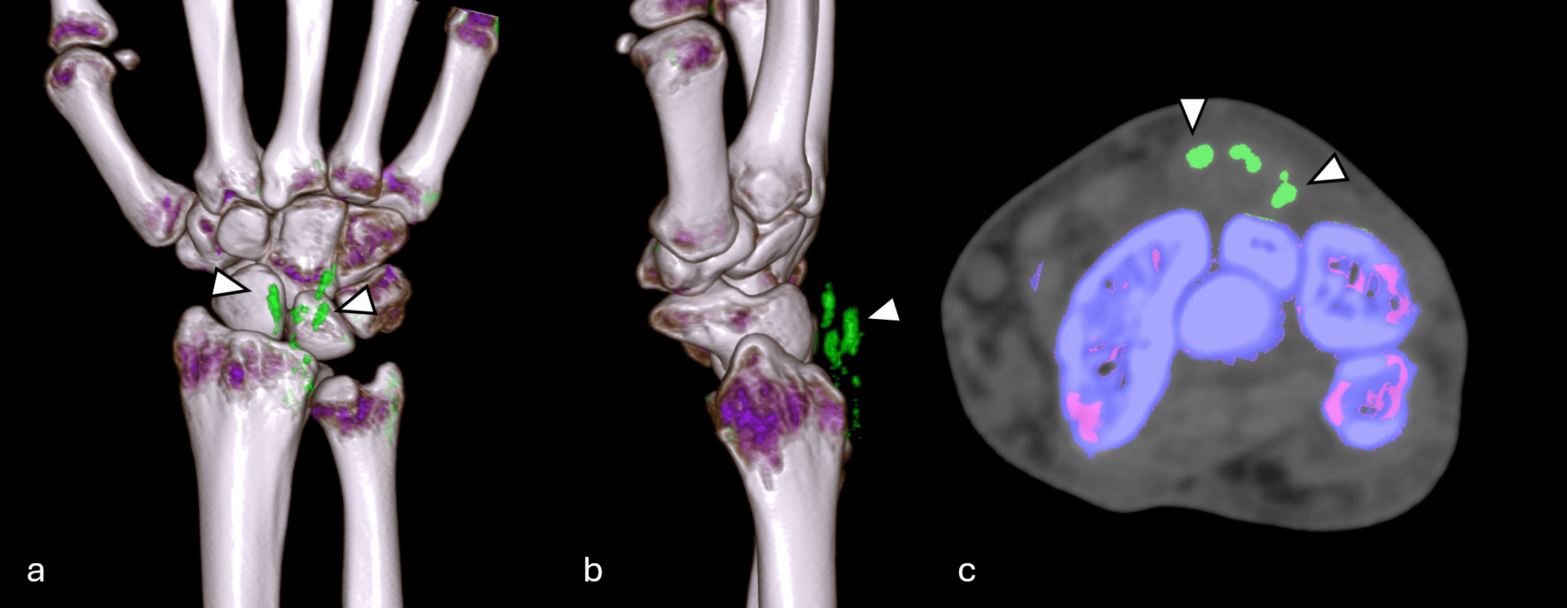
Dual-energy CT of the right wrist Dual-energy CT of the right wrist with 3D reconstruction (a, b) and at the axial plane (c). Dual-energy CT with color coding demonstrated the presence of uric acid deposits at the fourth extensor compartment of the wrist, consistent with gouty tophi (arrowheads) CT: computed tomography
